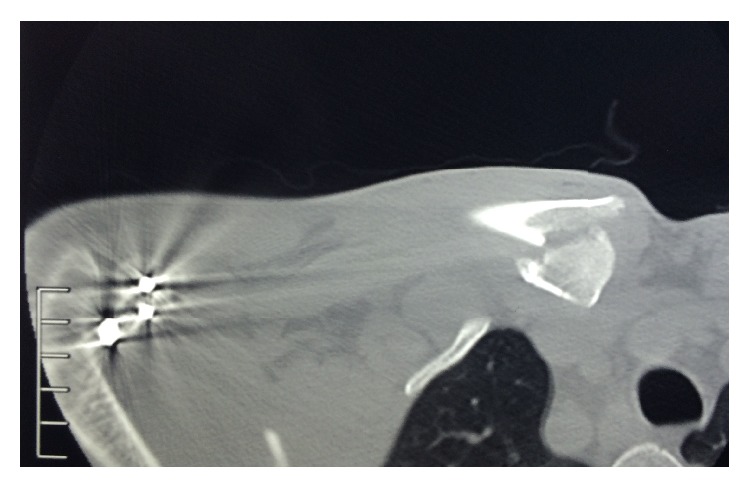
Medial clavicle fracture detected on CT scan.
